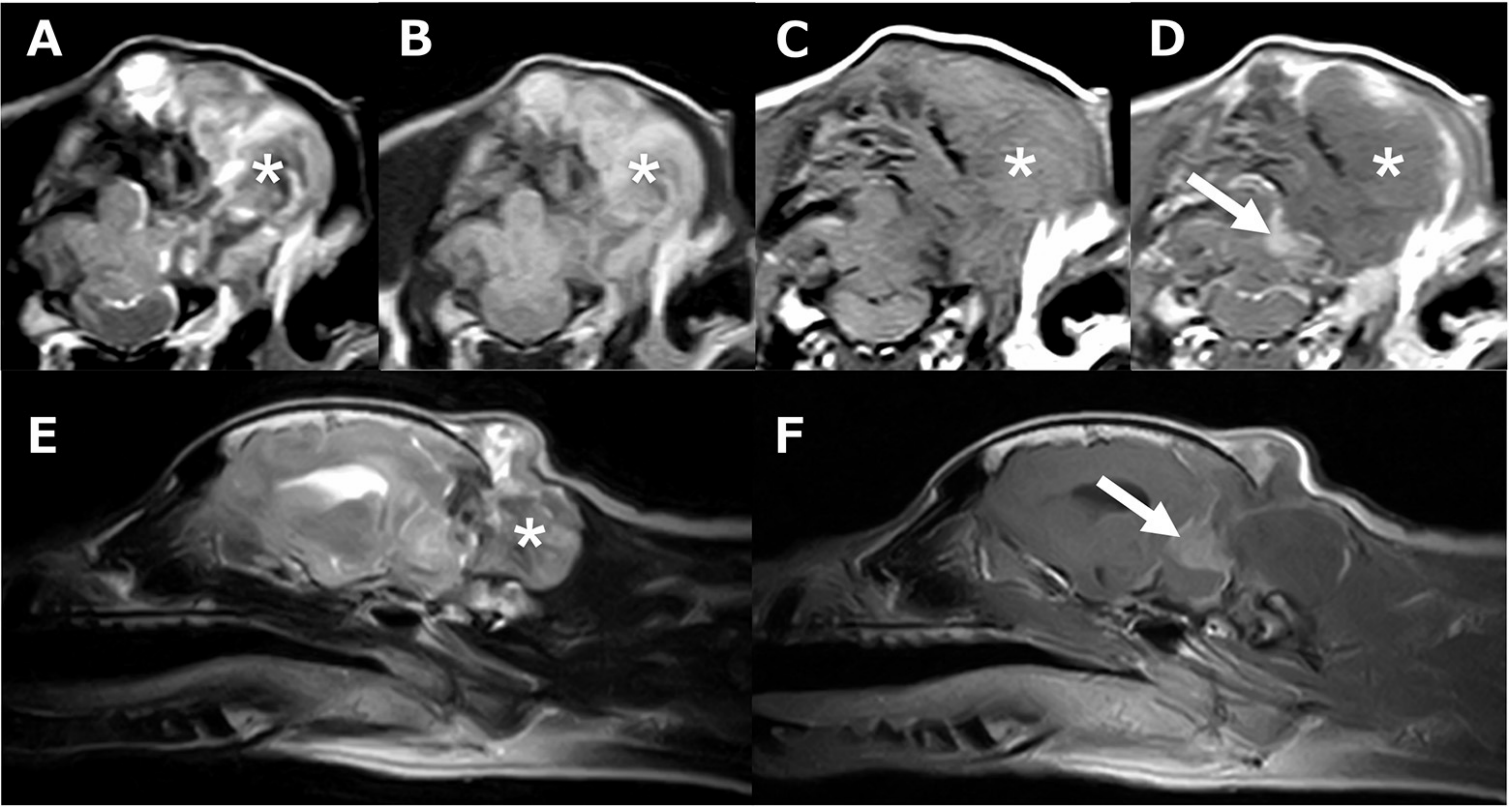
Head MR images. Transverse T2 weighted (A), FLAIR (B), T1 weighted (C), and T1 weighted post-contrast (D) images at the level of posterior fossa, and sagittal T2 weighted (E) and T1 weighted post-contrast (F) at the level of the left cerebellum. A large mass lesion was found in the left occipital subcutaneous tissue, posterior fossa, and left cerebellar bridge angle region. The mass showed mixed hyper intensity was recognized extracranial region (*) and strong enhancement on the margins and near the meninges (arrow).
